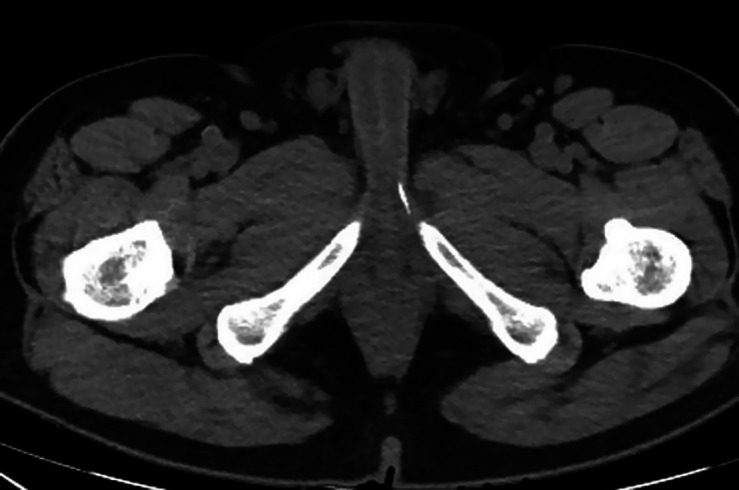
Computed tomography images showing single calcified Peyronie's plaques on left proximal sides of the penis.
